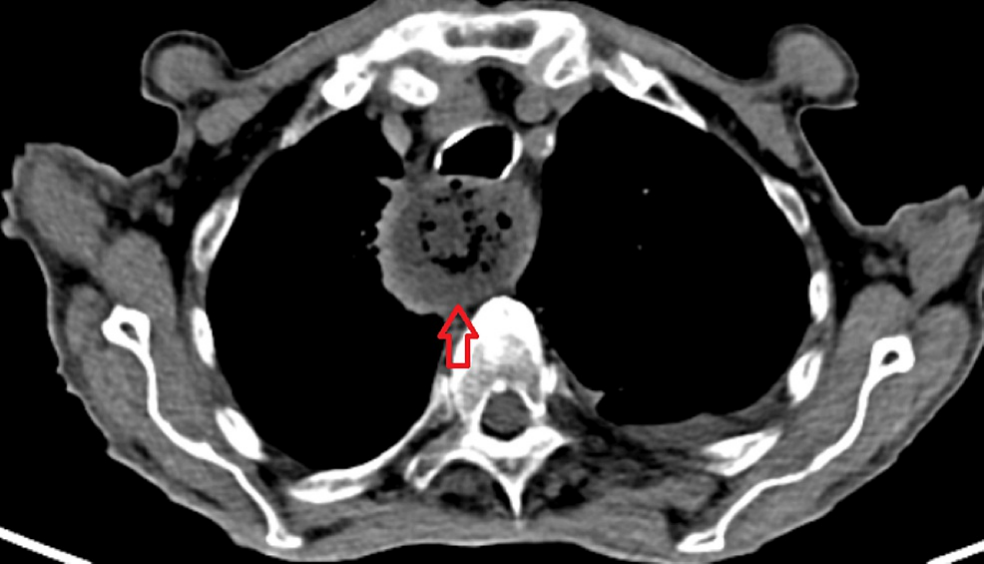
Thoracic CT scan Thoracic CT scan axial view revealed dilation of the thoracic esophagus (red arrow) with stenotic of the gastroesophageal junction
